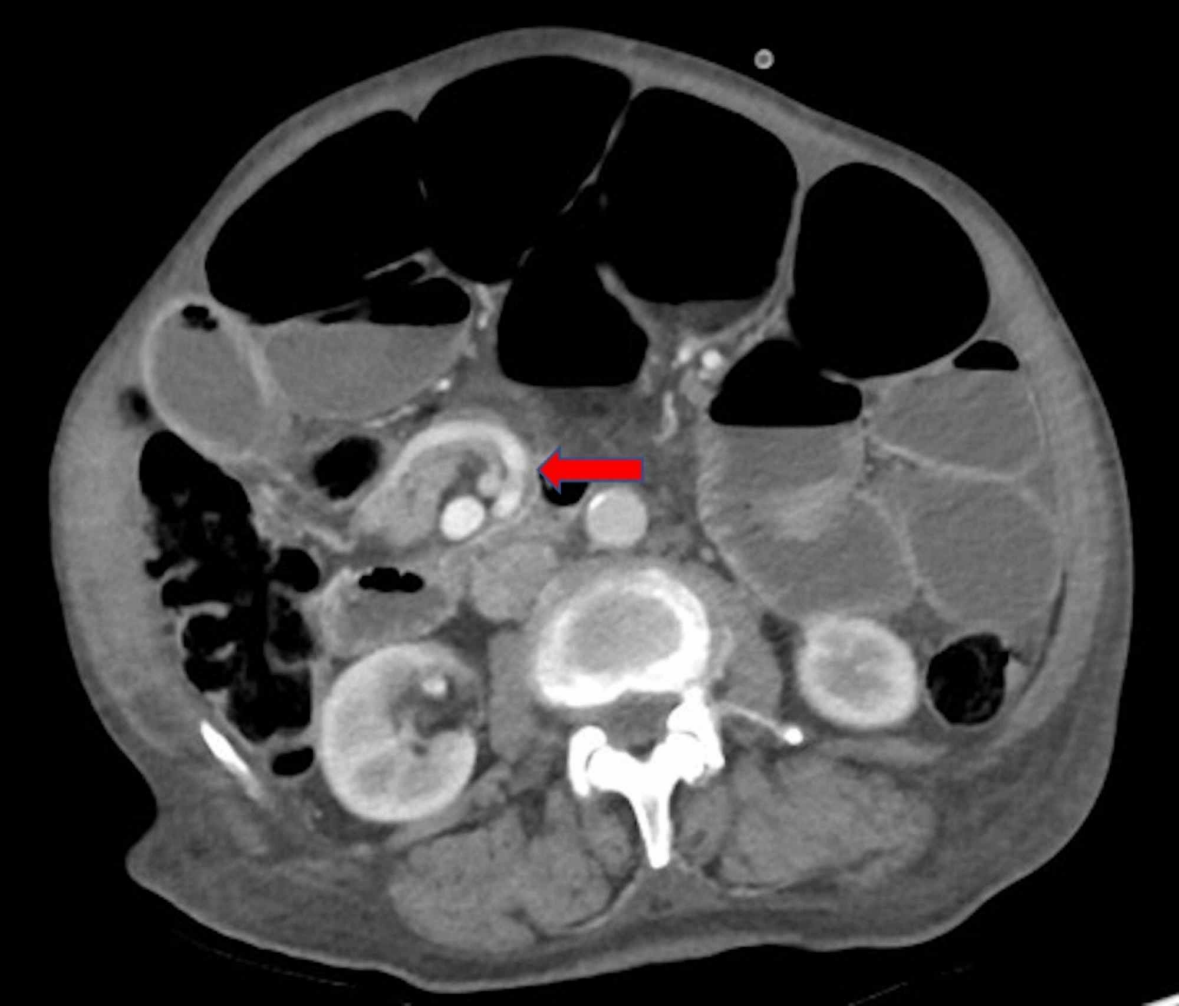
Axial slice of abdominal computed tomography (CT) after contrast media administration, in portal phase demonstrating “whirlpool sign” of mesenteric vessels, suggestive of small bowel volvulus (arrow) with dilated loops of small bowel
